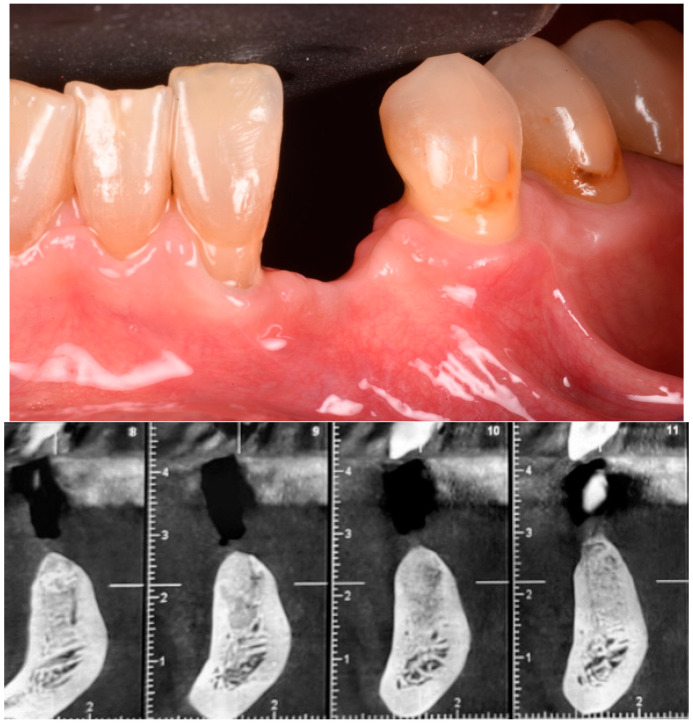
Soft tissue healing 8 months post-op and CBCT proving the shape of the ridge.
